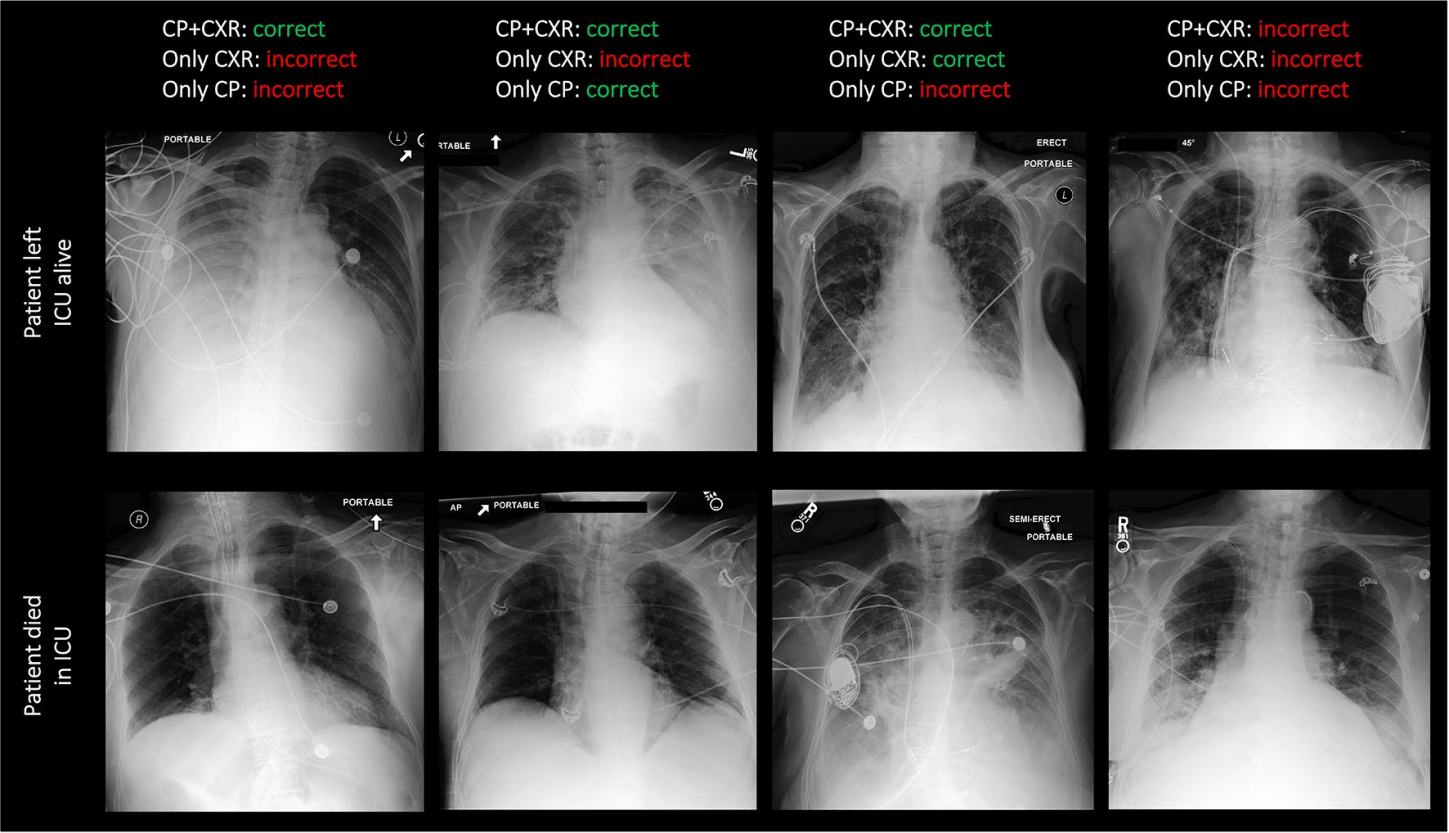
Exemplary chest radiographs and associated patient survival predictions. The upper row shows chest radiographs of four patients dismissed from the ICU alive. The lower row shows chest radiographs of four patients who died in intensive care. Predictions of the model were
